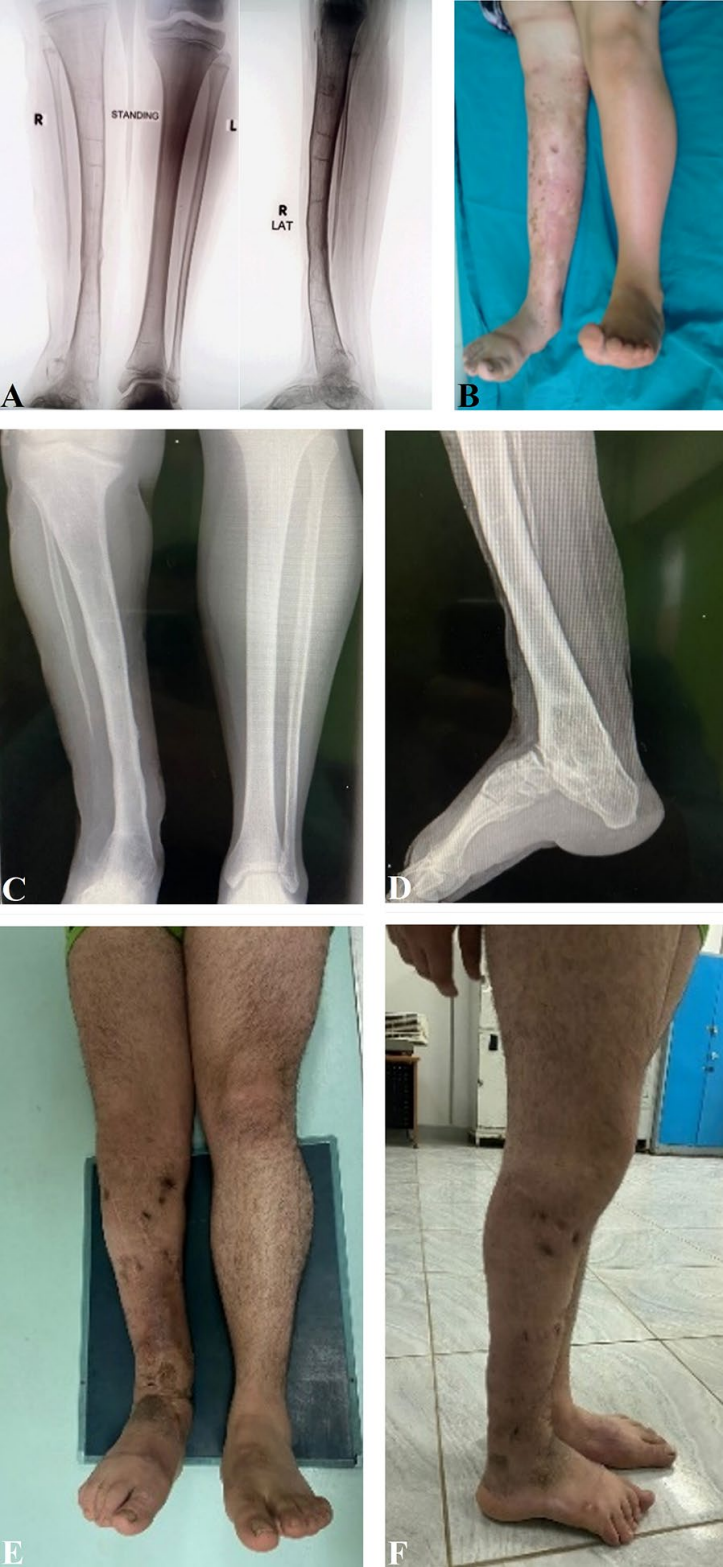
Follow-up X-rays and clinical photographs of the same patient. A Five years’ follow-up AP and lateral X-rays showing overgrowth of the tibia by 4 cm together with valgus ankle. B Five years’ follow-up clinical photographs. C Fifteen years’ follow-up AP view showing varus deformity at the knee with depression of the medial joint space. There is also valgus deformity at the ankle with 1.5 cm shortening. D Lateral X-ray of the leg and ankle shows total fusion of the ankle and subtalar joint and an increased calcaneal pitch angle. E, F Clinical photographs of the patient when he became 21 years old
